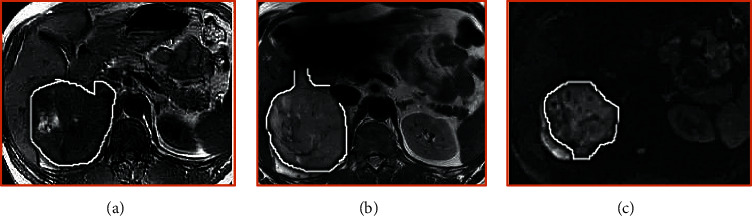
Adrenocortical tumors. (a) T1WI. (b) T2WI. (c) DWI.
